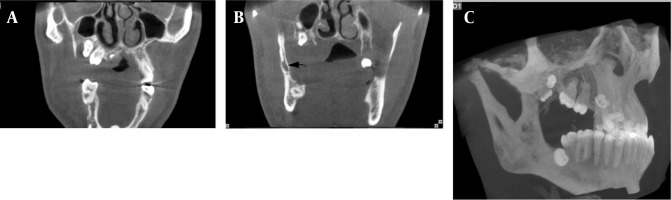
A and B, Coronal CBCT images of the same case reveal expansile mass on the right side of the maxilla with elevation of right antral floor, soft tissue bulging in palatal portion and lucent cystlike lesion in inferior portion of right ramus with thinning and expansion of cortical portion; C, 3D reconstructed image showing gross deformity of maxilla and mandible on the right side.
